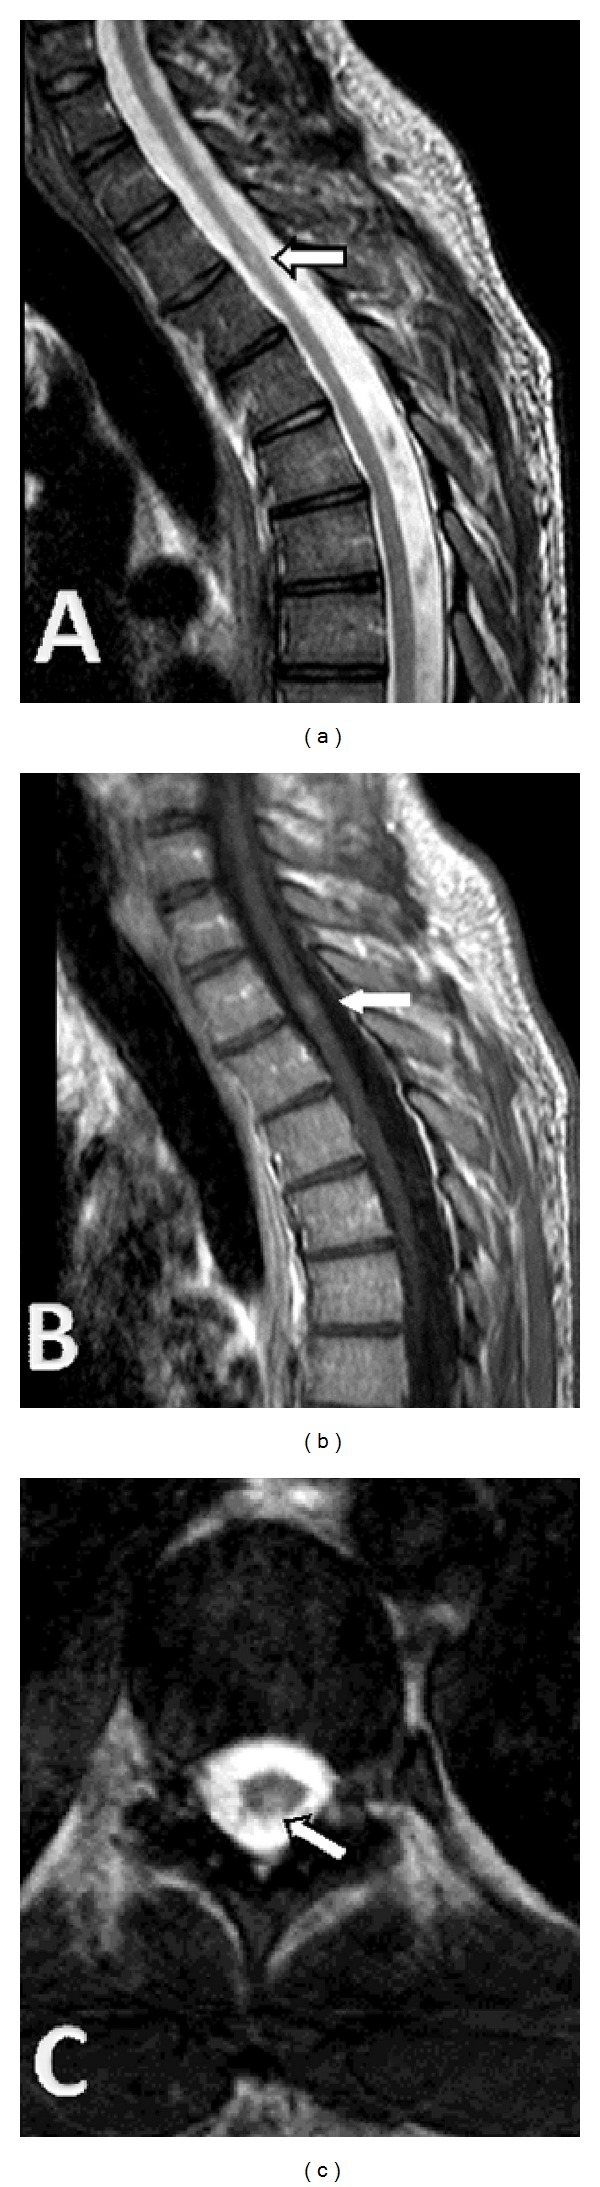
Thoracic spinal cord MRI at second attack. (a) Sagittal T2-weighted image showing a hyperintense lesion at T3 level. (b) Sagittal T1-weighted image showing gadolinium-enhancement of the lesion at T3 level. (c) Axial T2-weighted image showing a hyperintense lesion at T11 level.
